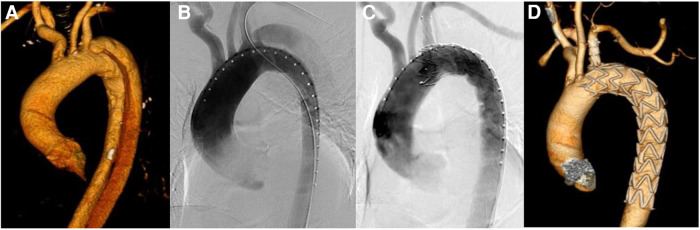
Results of aortic imaging in patients at different periods. (A) Is the preoperative CTA image of aorta. (B) Is the result of DSA angiography of aorta during operation, and the intimal rupture is visible near the root of LSA. (C) Is the result of DSA after the release of Castor integrated branched aortic covered stent. The intimal rupture is closed, with no visible endoleak, and LSA is unobstructed. (D) Is CTA imaging review of aorta more than one month after operation, which shows that the stent is well extended, the original false lumen is thrombed and LSA is unobstructed.
